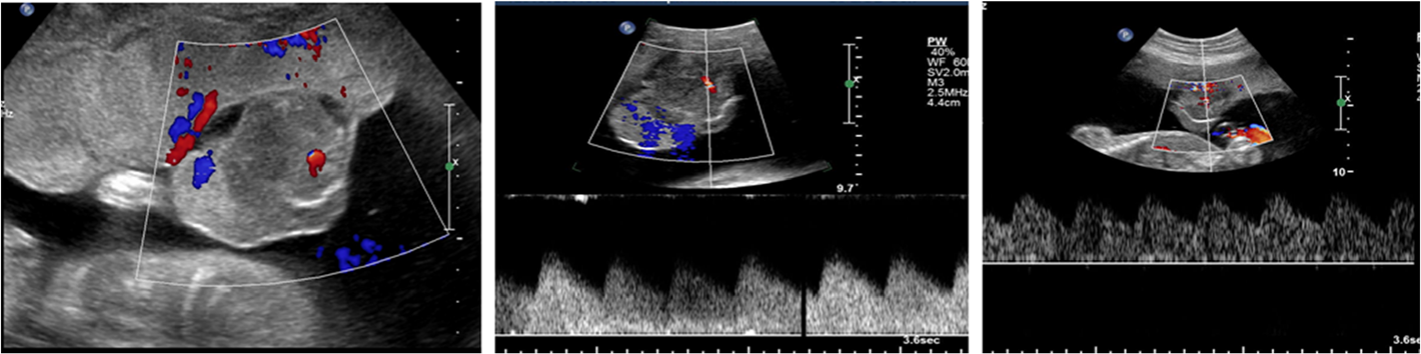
Ultrasound image of the highly vascularized chorioangioma.
